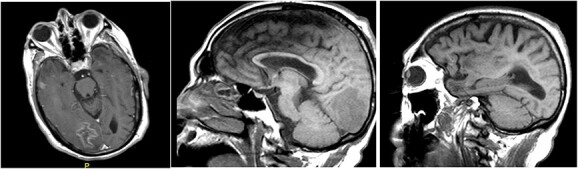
MRI of brain, with and without contrast, identified four enhancing lesions, concerning for intracranial metastatic disease, located in the right temporal (left), occipital (center) and frontal lobes (right).
